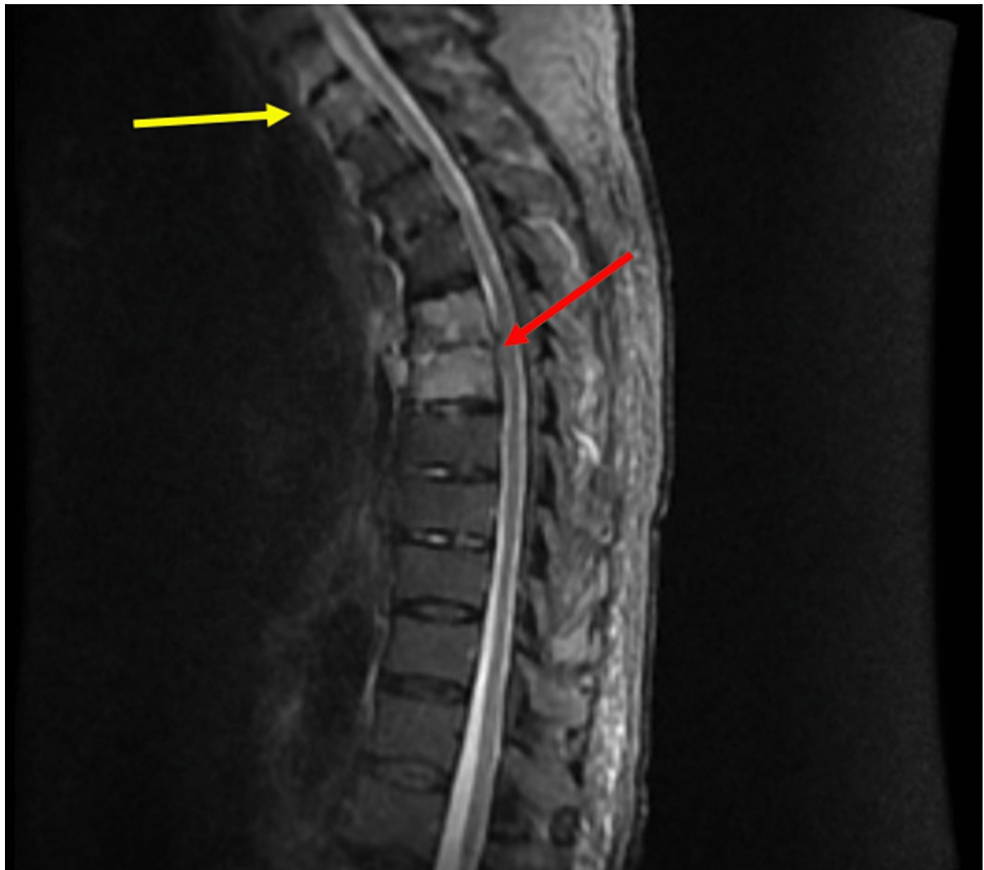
Magnetic resonance imaging of thoracic spine showing osteomyelitis/discitis at T6-T7 (red arrow) and likely osteomyelitis at T1 and T2 (yellow arrow).
